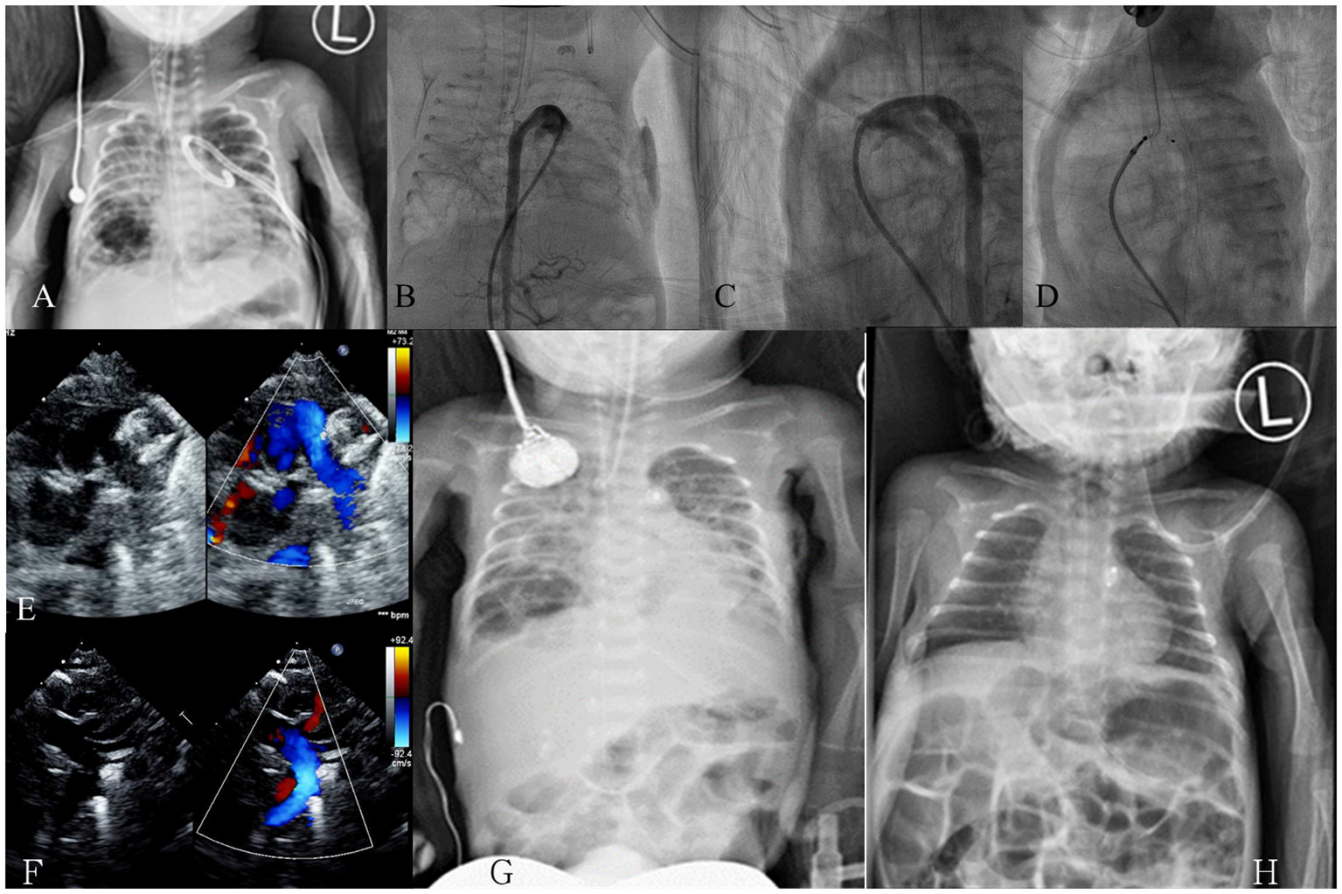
Case demonstration in a premature baby with a gestational age of 26 ± 3 weeks and a birth body weight of 766 g. (A) Chest X-ray on day 34 revealed poor lung condition with a left side pneumothorax post pigtail catheter; (B) On day 36 (body weight, 836 g), an anteroposterior view angiogram revealed a large tubular PDA; (C) Lateral view angiogram revealed a tubular type-C PDA, size: 2.9 mm, length: 6.4 mm; (D) An ADO-II-AS (4 × 4 mm) device was deployed on day 36; (E) Echocardiogram revealed device in position without pulmonary artery obstruction; (F) Echocardiogram revealed no evidence of coarctation of the aorta; (G) Follow-up chest X-ray on day 38 (2 days after the procedure) revealed rapid improvement; (H) Follow-up chest X-ray on day 70 (34 days after the procedure) revealed favorable condition. ADO-II-AS, Amplatzer Duct Occluder II with additional size; PDA, patent ductus arteriosus.
